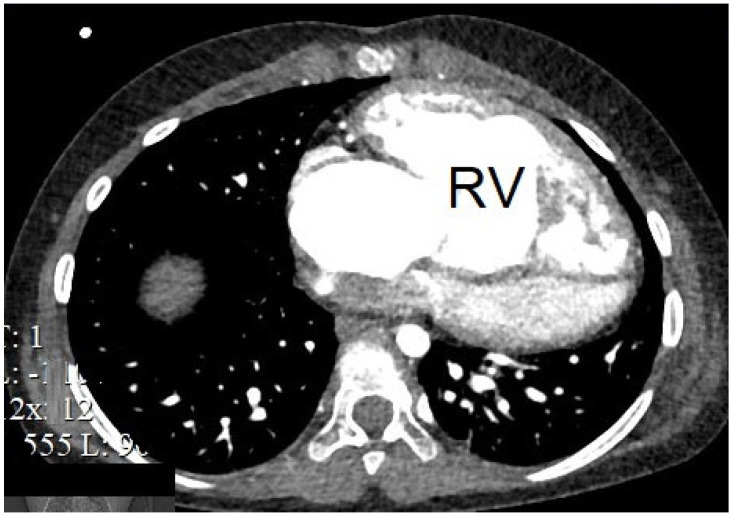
A computed tomography scan showed a dilated systemic right ventricle (RV) in a child after Mustard procedure.
